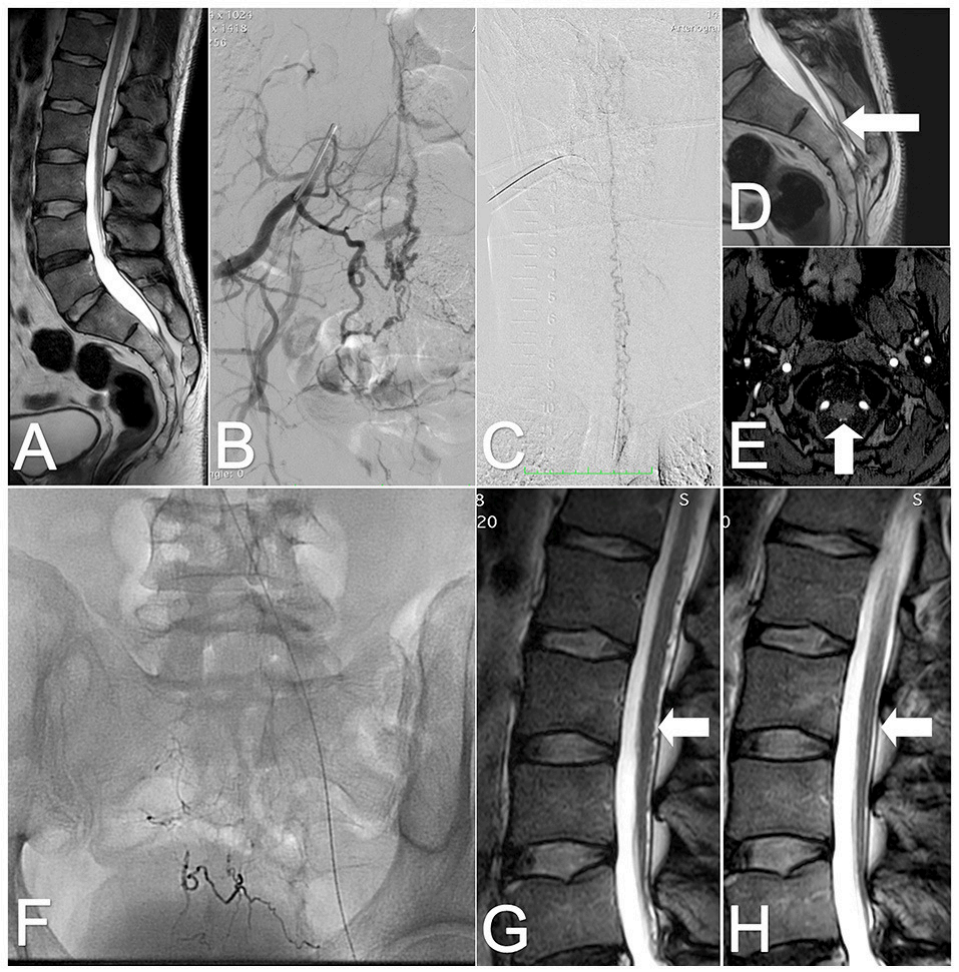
Images of the spinal cord of case 2, before and after treatment. (A) T2-weighted MRI: Tethered cord and sacral lipoma. (B) DSA: SDAVF at the S2-S3 level supplied by the lateral sacral arteries. (C) DSA: Tortuous veins on the posterior surface of the cervical spinal cord. (D) T2-weighted MRI: Tortuous vein on the posterior surface of the tethered spinal cord (arrow). (E) TOF MRI: Draining vein (arrow) at the atlanto–occipital junction level. (F) X-ray of the sacral area shows embolic agents after three embolizations. (G) Lumbar T2-weighted MRI: After the last embolization, tortuous vessels are visible on the posterior surface of the spinal cord (arrow). (H) Follow-up MRI: at 6 months after the surgery, the tortuous veins are no longer observed on the posterior surface of the spinal cord (arrow).
